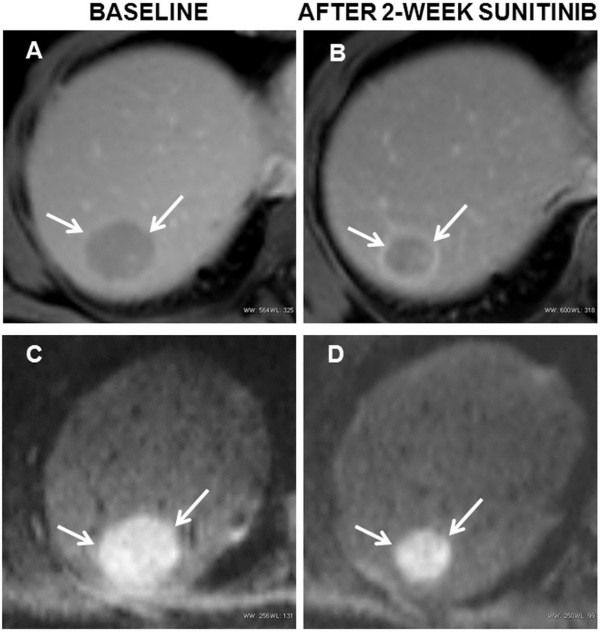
MR diffusion weighted images during sunitinib therapy. Transverse enhanced MR T1-weighted images (A, B) and MR diffusion weighted images (DWIs) for estimated apparent diffusion coefficient (ADC) (C, D) at baseline and after 2-week of sunitinib therapy in the same 47-year-old man with HCC (arrows). After two weeks of treatment with sunitinib, there was an increase in ADC value from 0.86 × 10-3 mm2/s to 0.94 × 10-3 mm2/s.
